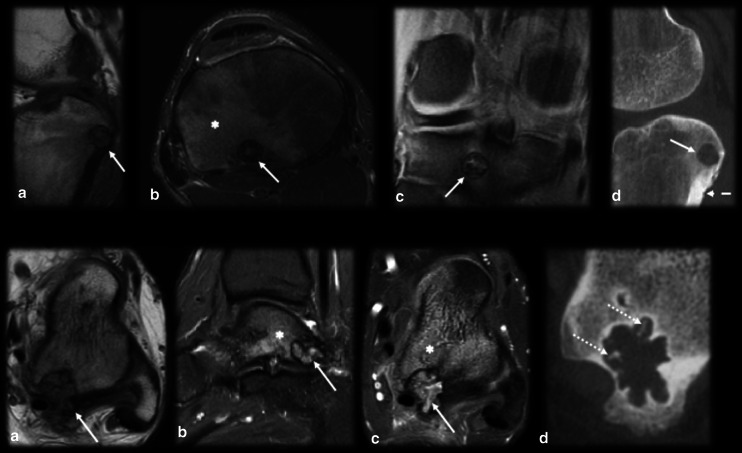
Osteoid osteoma versus chondroblastoma. (a–d) Male, 29 years old, presenting with knee pain for 3 months. MRI sagittal T1 (a), axial and coronal T2 FS (b, c) and CT (d) showed a round and central mineralized nidus (arrows) with reactional bone marrow edema (asterisk) and cortical thickening (dashed arrow in d). (e–h) Male, 24 years old, presenting with ankle pain for 9 months. MRI axial T1 (e), sagittal T2 (f), axial T1 FS GD (g) and CT (h) showed a large and lobulated bone lesion (arrows) with peripheral arciform calcifications (dotted arrows), internal enhancement (c) and reactional bone marrow edema (asterisk)
